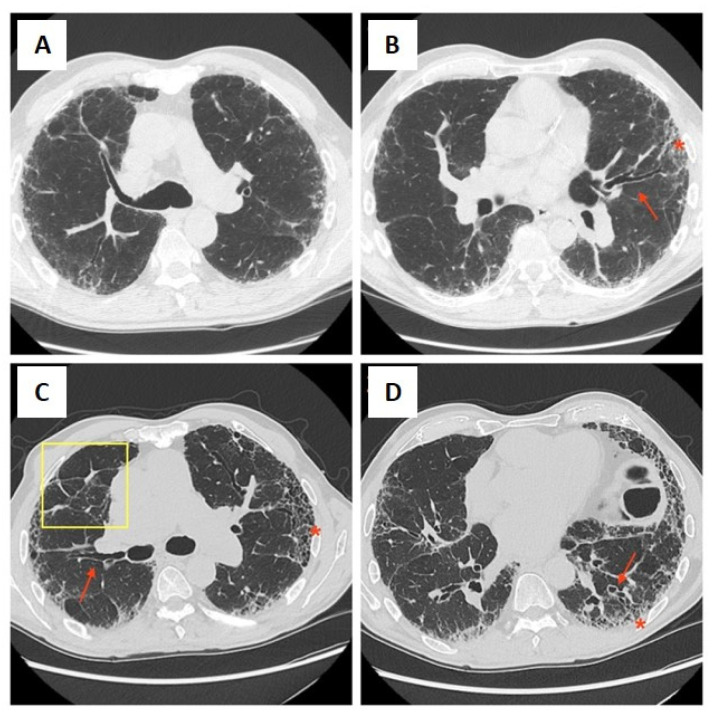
CT lung scan of a 75-year-old male patient at the time of UIP diagnosis at our center, after Pirfenidone was started. The CT scan in boxes (A,B) show traction bronchiectasis (red arrow) and honeycombing areas mainly located at the basal zone (red asterisk). CT images in boxes (C,D) were acquired after 3 years of follow up and specific therapy and show progression in findings already present (red arrow and asterisk) together with septal thickening (yellow square), another UIP radiological feature.
